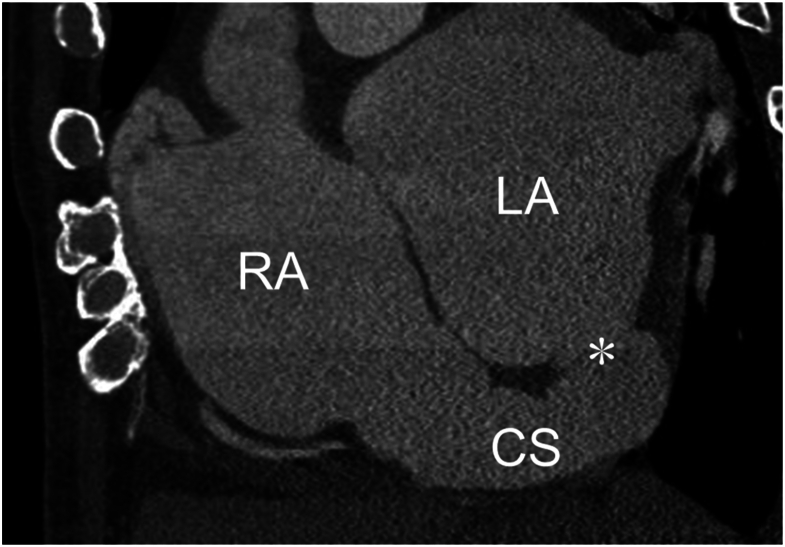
Preoperative cardiac computed tomography scan showing a dilated coronary sinus communicating with the left atrium. ∗Unroofed portion of the coronary sinus. (CS, coronary sinus; LA, left atrium; RA, right atrium.)
